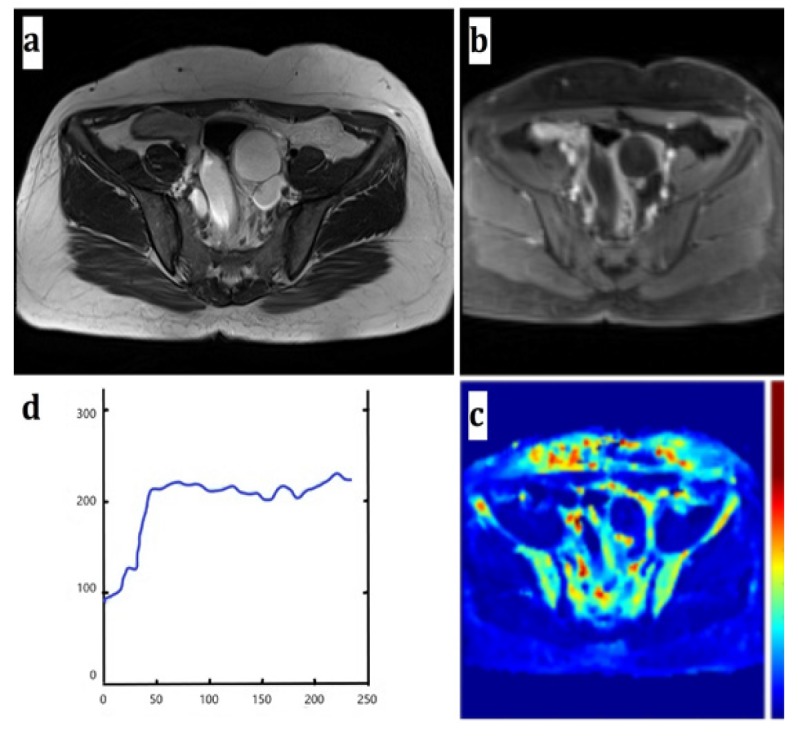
Figure 1Region of Interest (ROI) drawn on a benign adnexal mass (a serous cystadenoma) (a) Axial T2-weighted image (b) Axial DCE T1-weighted image (c) Ktrans coded color map, ROI was drawn on a small enhancing papillary projection of the tumor. (d) Benign enhancement curve and pharmacokinetic and semi-quantitative parameters
